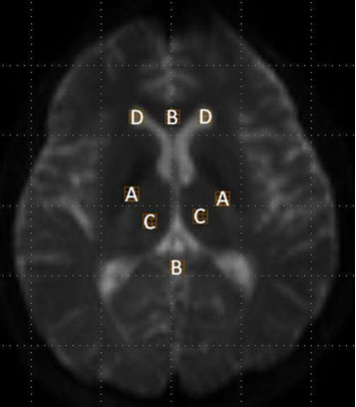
Superimposed volumes of interest on T2-weighted image without motion probing gradient. *A* Posterior limb of the internal capsule, *B* corpus callosum, *C* thalamus, *D* anterior horn of the lateral ventricle
